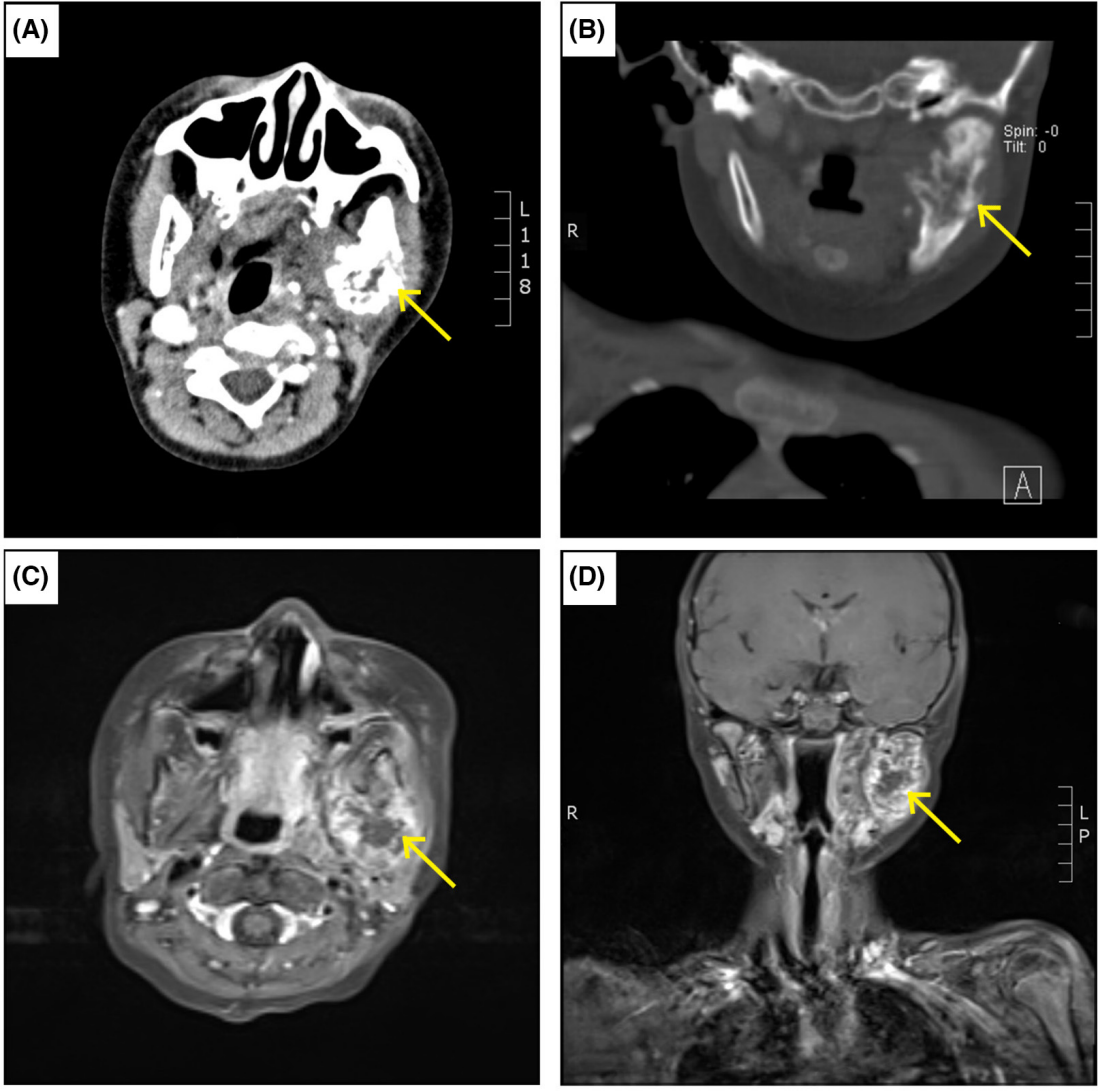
Radiographic images of the yolk sac tumor patient. (A, B) Computed tomography (CT) showed a left facial mass (Yellow arrow) with transverse (A) and coronal section (B). (C, D) Magnetic resonance imaging (MRI) displaced an abnormal signal mass in the left facial mass (arrow) with transverse (C) and coronal section (D).
